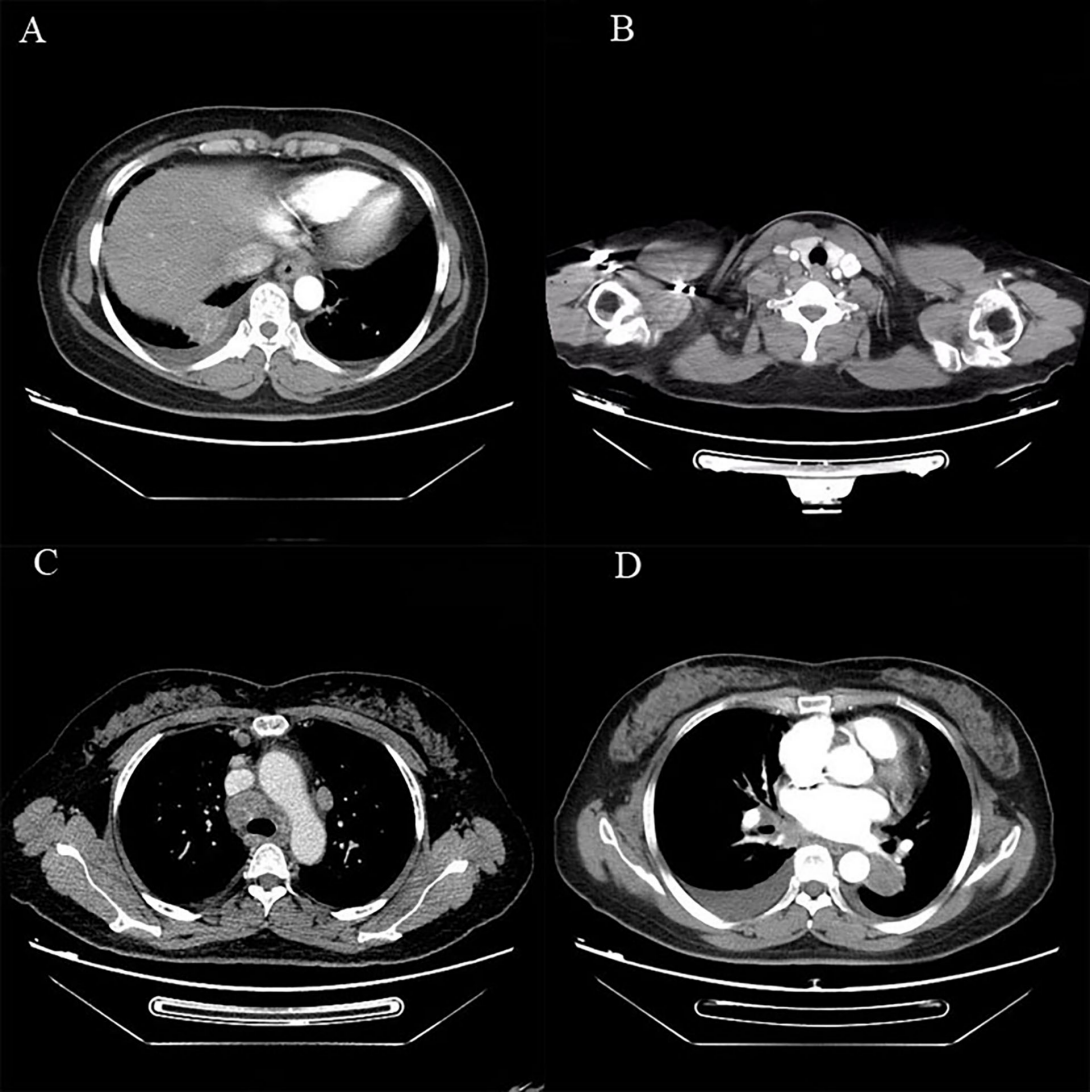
Chest CT of the patient before treatment: (A–D) show the range of lesions, respectively.
